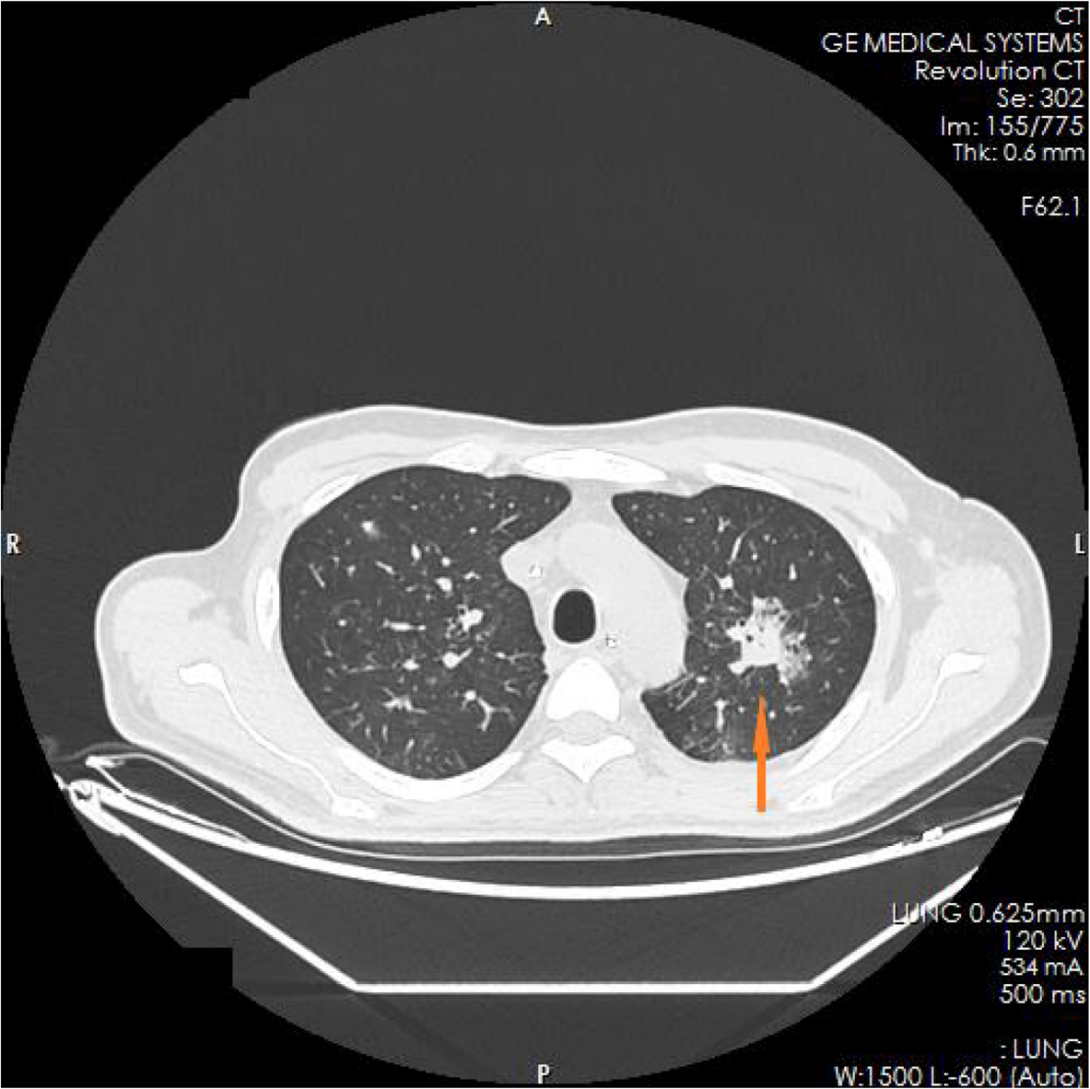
CT pictures displaying multiple tuberculosis lesions throughout the body
